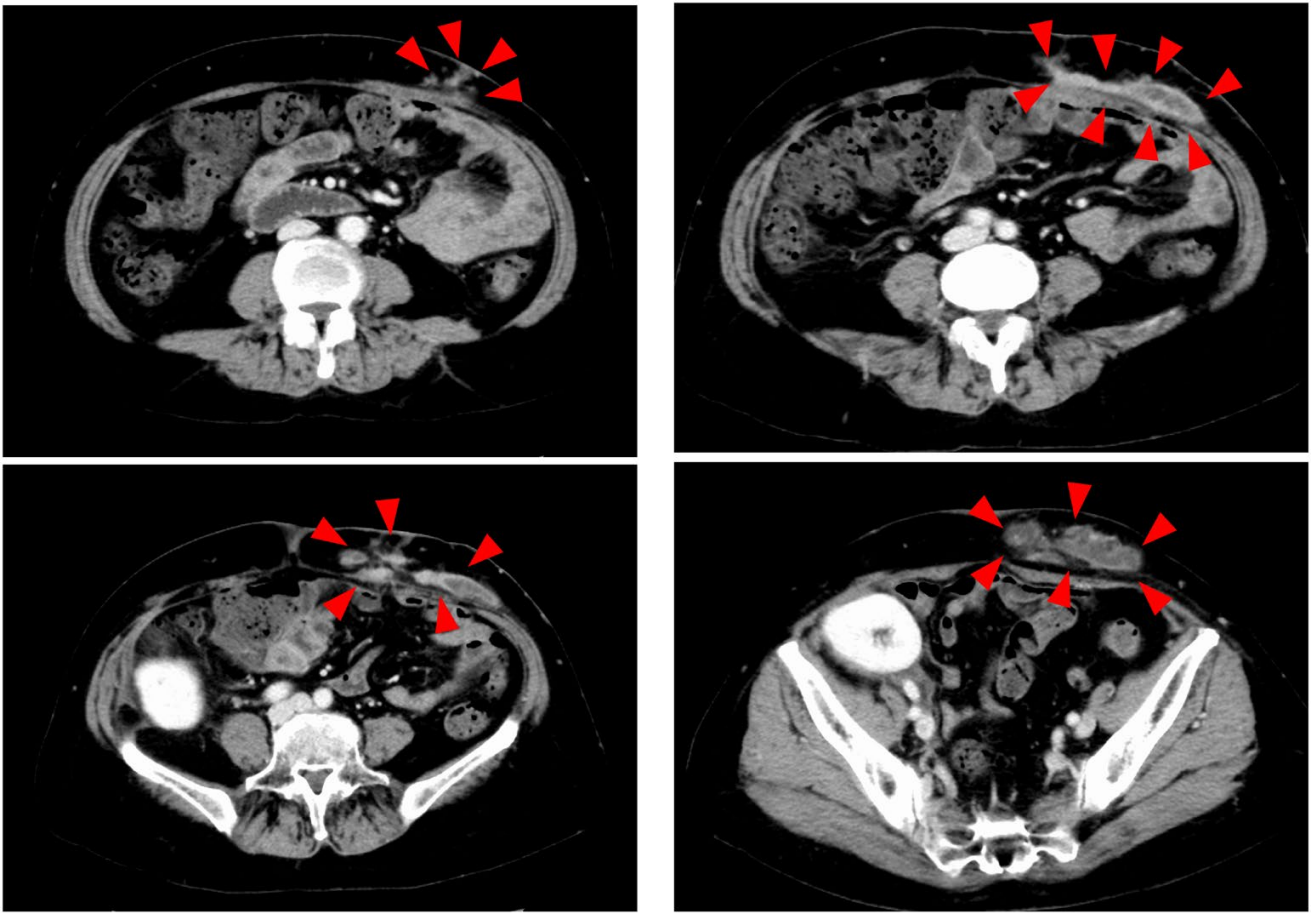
A CT scan revealed a widespread abscess at the site of the PD catheter removal.
